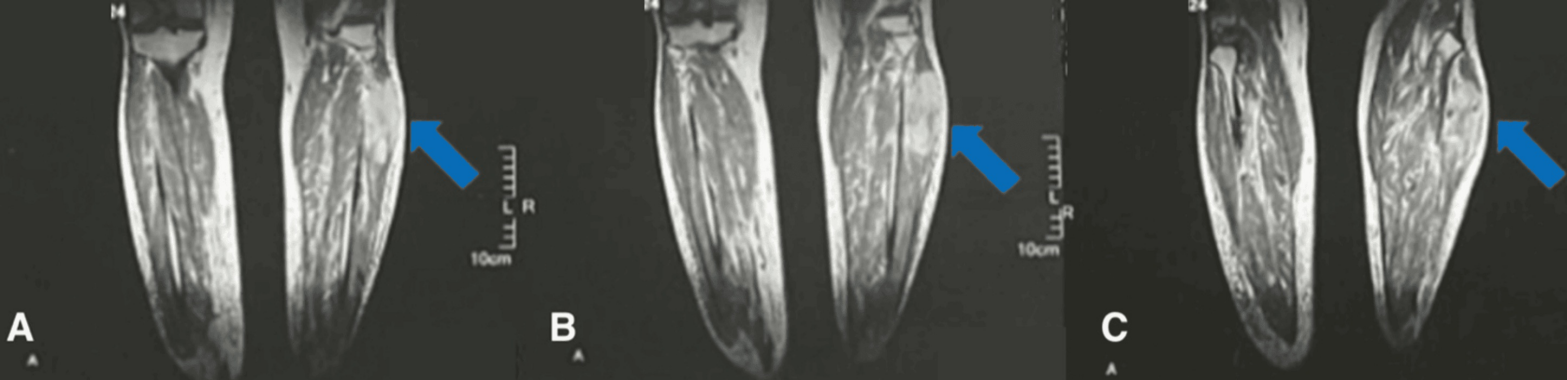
MRI of the left leg in coronal T2-weighted sequence showing a fusiform, heterogeneous mass, measuring 165 × 40 × 50 mm, centered on the fibular muscles in the upper third of the left leg (arrow). (A-C) Various coronal sections demonstrating a fusiform mass centered on the fibular muscles.
